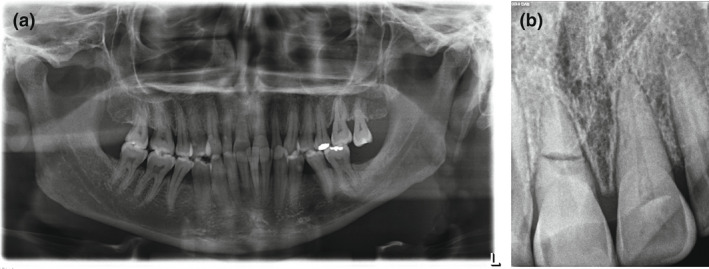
(A) Panoramic image of the same patient taken by a dental student using the simple breathing technique instruction. The teeth are over‐closed as patient refused to open the mouth. Tooth 11 (right central incisor) apex is not visible, indicating a need for a single intra‐oral radiograph. (B) Periapical image of tooth 11 (right central incisor). An occlusal technique was tolerated by the patient. A root fracture tooth 11 is revealed
